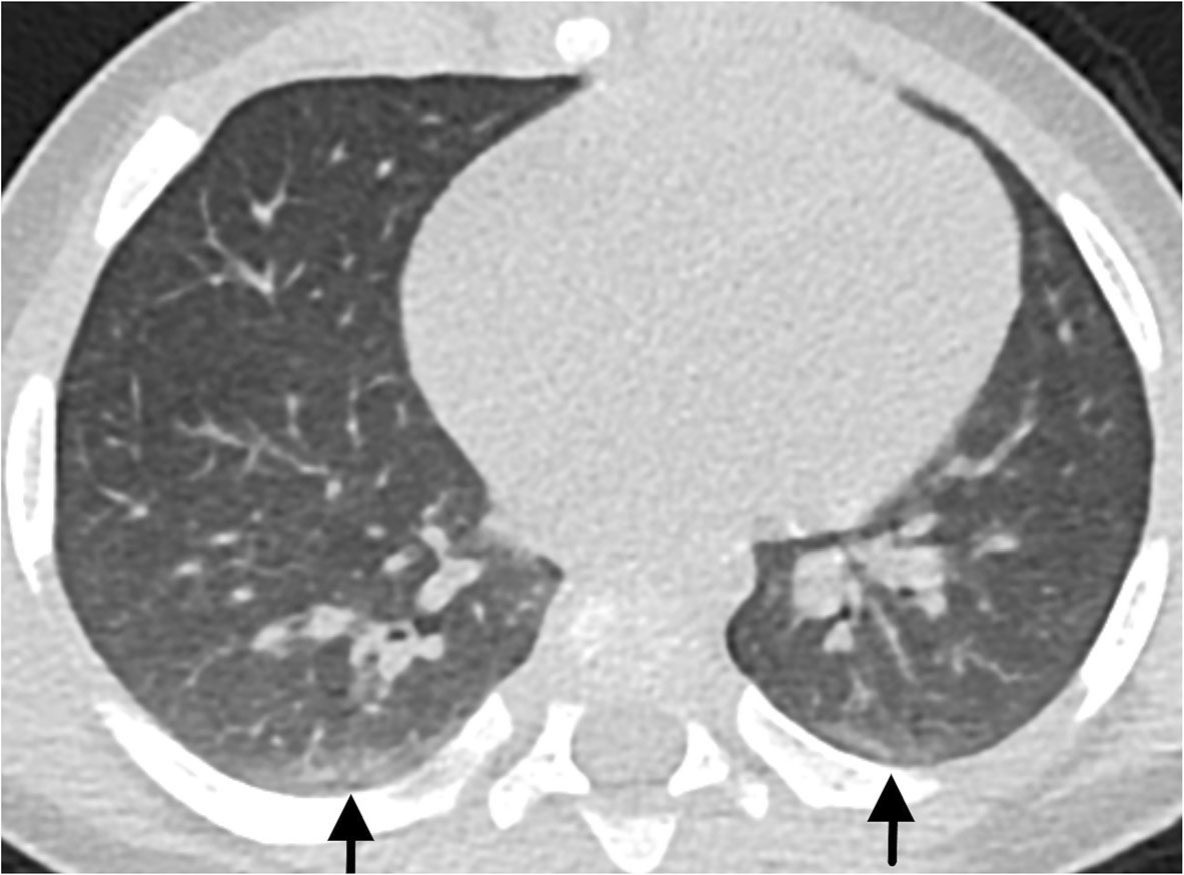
Normal/negative CT findings in a 7-month-old male—linear subpleural opacities in the posterior lungs (black arrows), commonly documented in the dependent position of the lungs of normal patients (due to athelectasic bands caused by hypoventilation/sedation)
